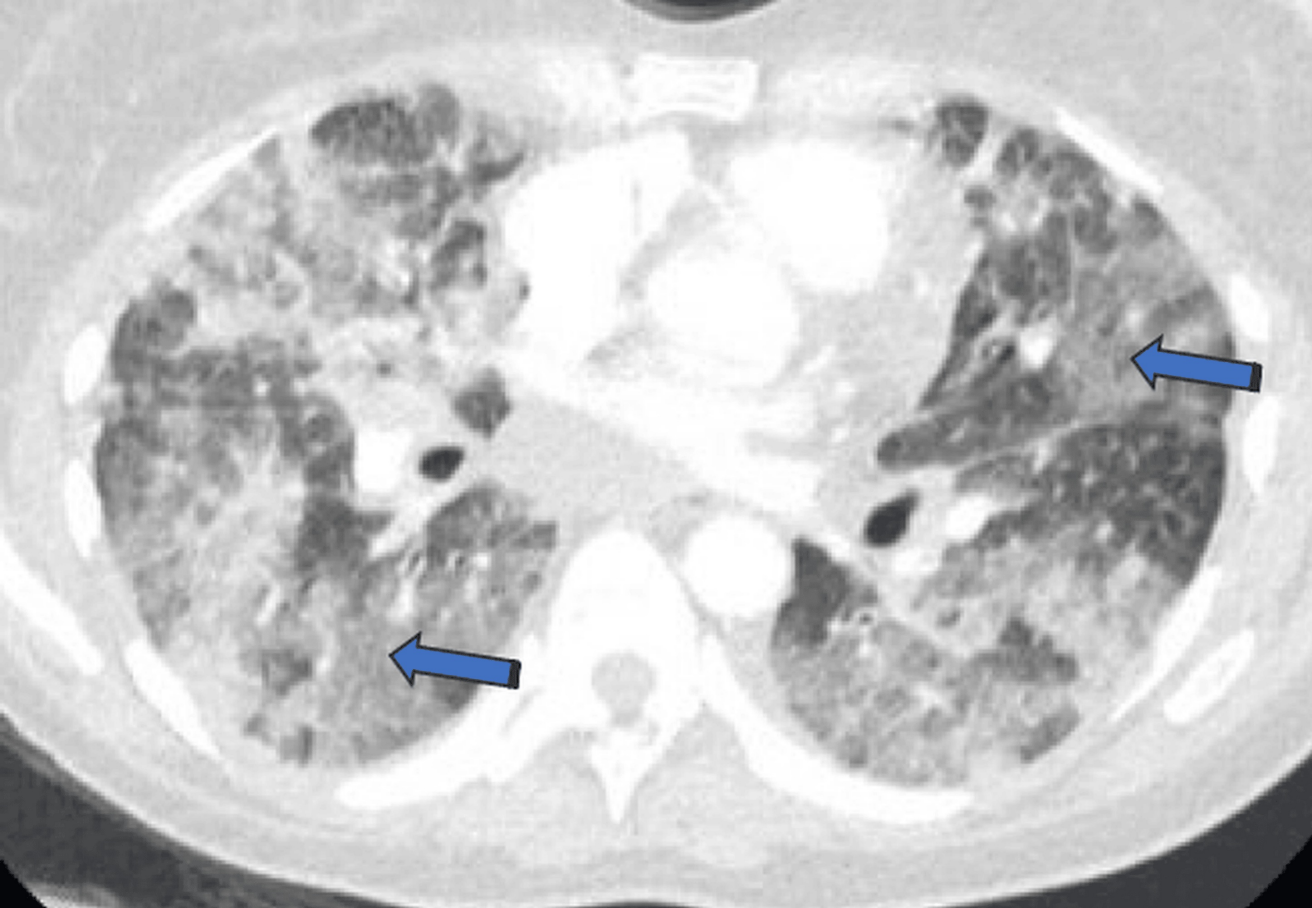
Computed tomography of the chest demonstrating diffuse lower lobe-predominant ground-glass opacities (blue arrows)
